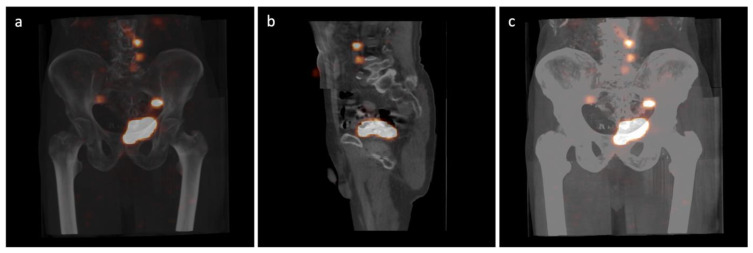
Sentinel lymph node (SLN) procedure in a bladder cancer patient. Fused SPECT-CT images to facilitate the anatomical identification of the SLNs: (a) frontal exposition; (b) sagittal exposition; (c) skeletal filtering.
